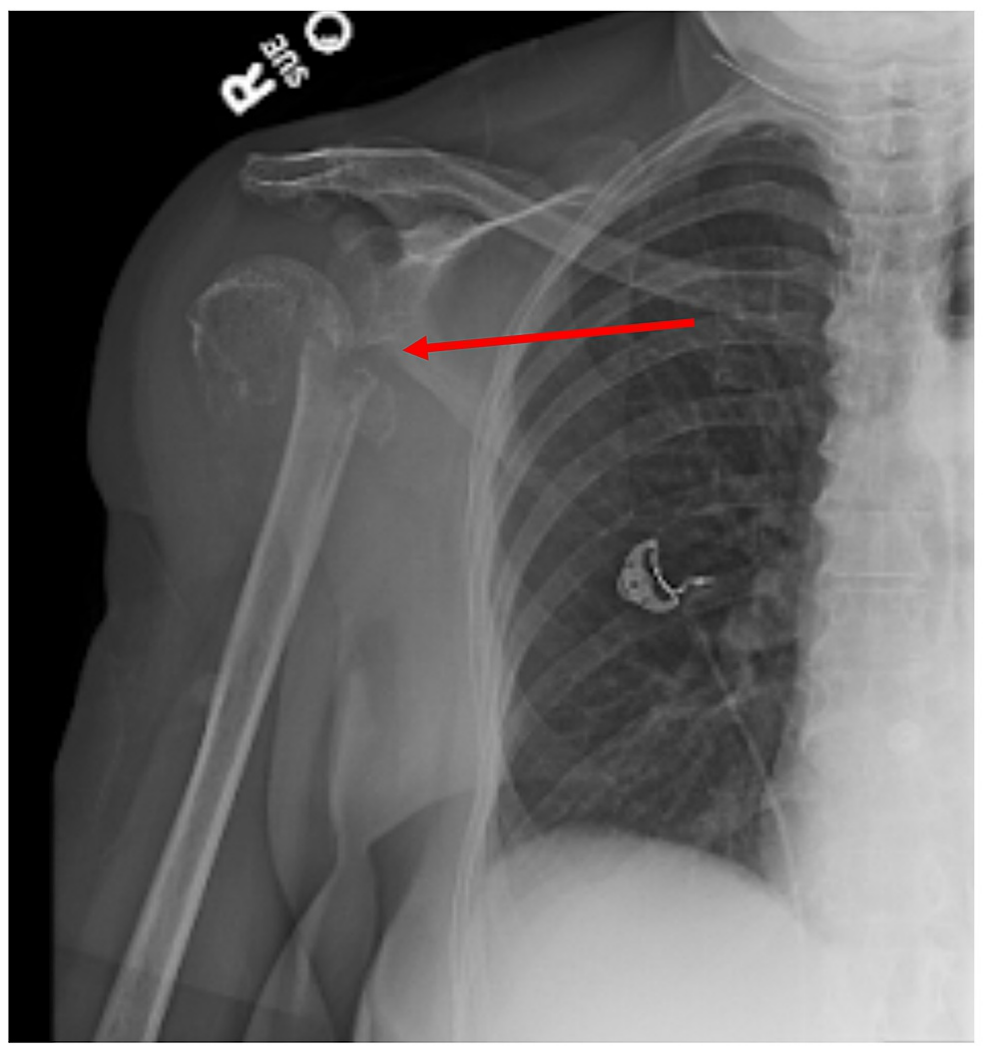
Right upper extremity X-ray depicting a right humeral neck fracture (red arrow)
